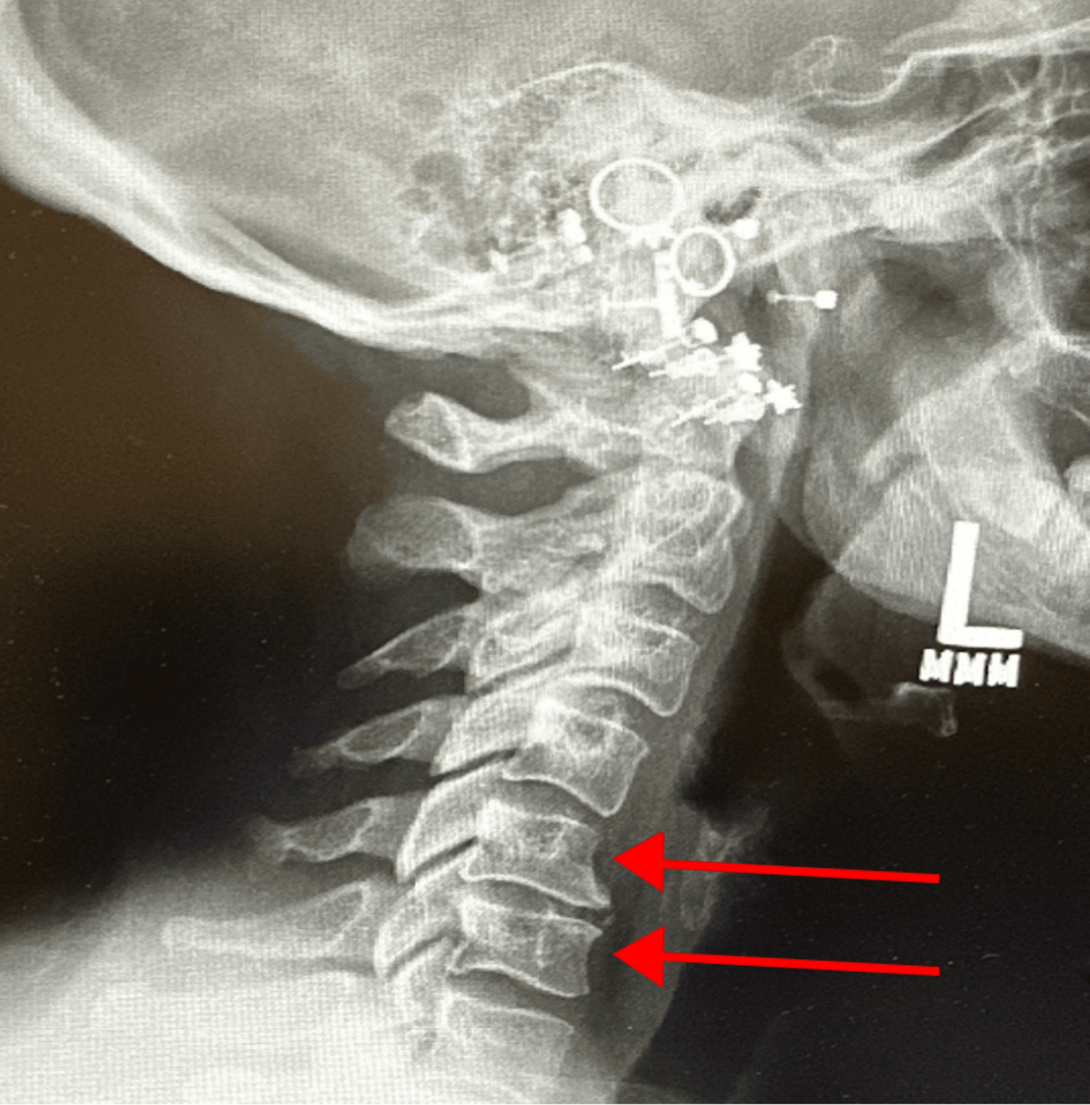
Lateral cervical radiograph highlighting C5–C6 degenerative disc disease and reversal of lordosis (red arrows) L: Left
